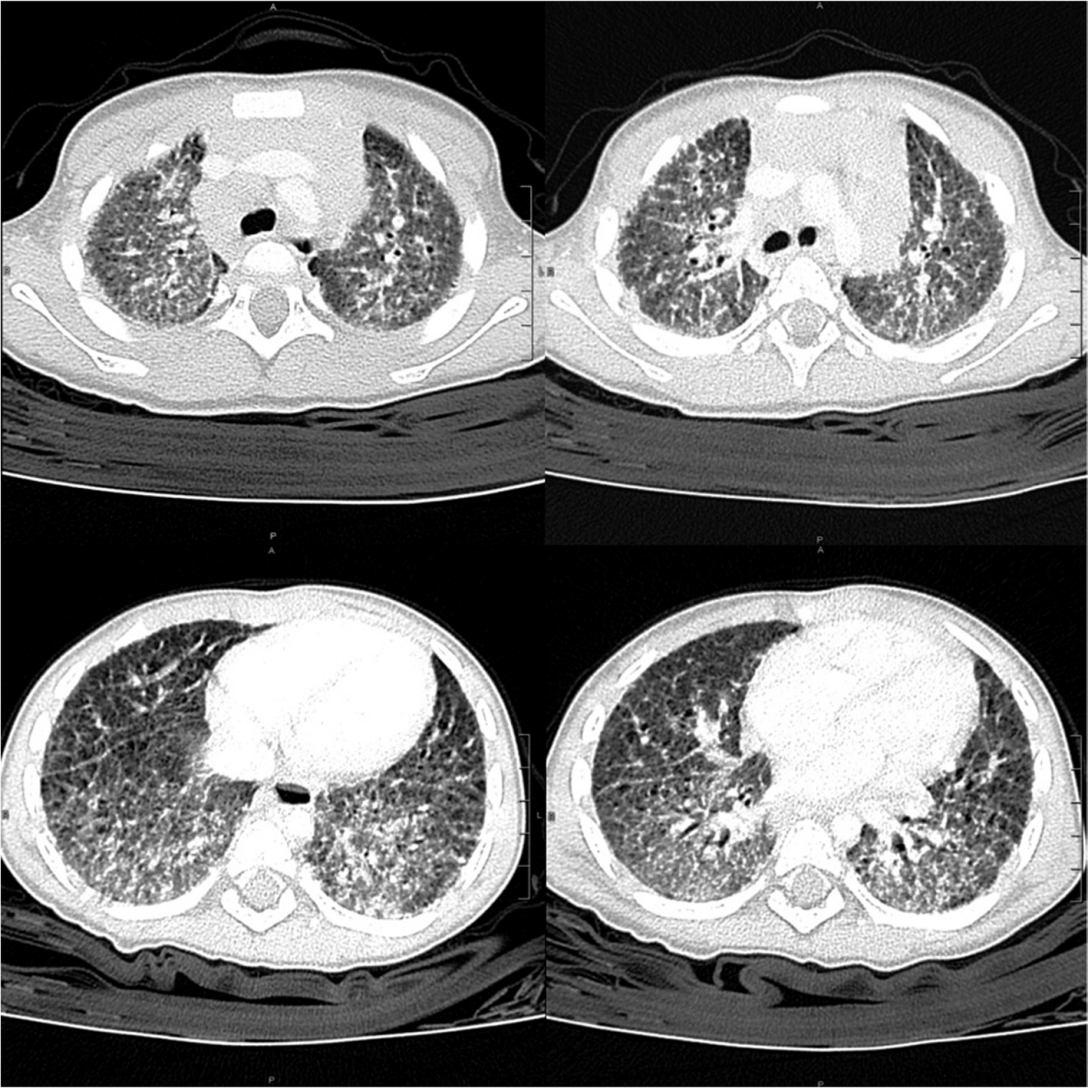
Chest CT scan shows diffuse marked thickening of the pulmonary interstitium with ground-glass areas, especially at the lower lobes. Bilaterally, numerous tiny cystic formations associated with bronchiectasis are evident at the subpleural level. Massive lymph node swelling in all mediastinal stations is present
